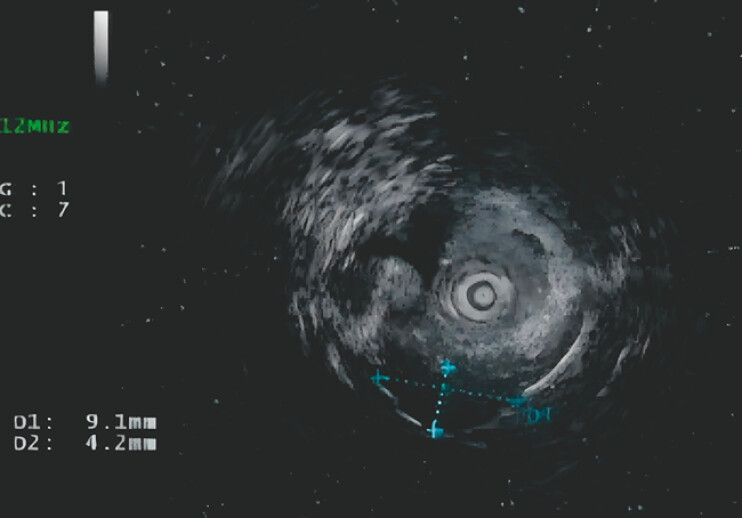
Ultrasound colonoscopy showed a submucosal bulge with a maximum cross-section of approximately 9.1 mm × 4.2 mm.
